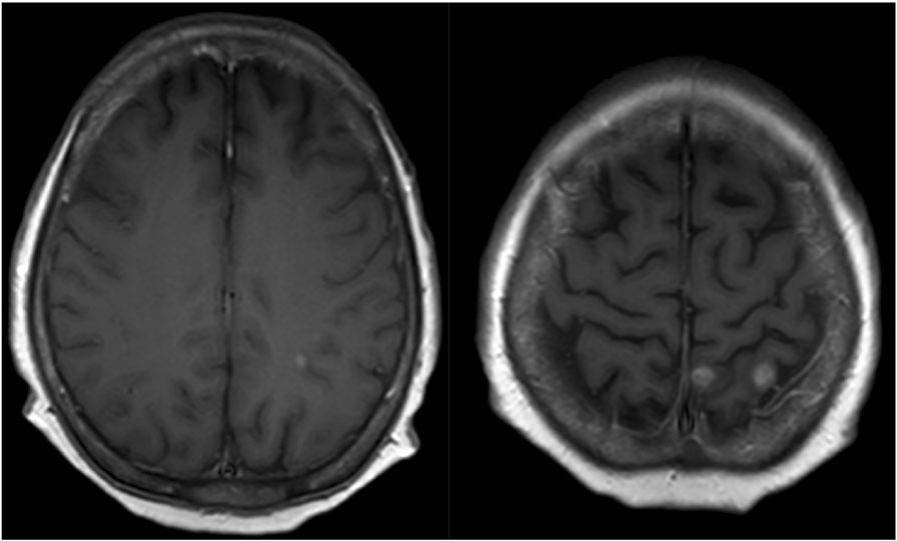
Multiple small gadolinium enhancing lesions with adjacent vasogenic edema at the subcortical region of the left frontal and parietal areas shown on T1W axial images of the pituitary MRI
